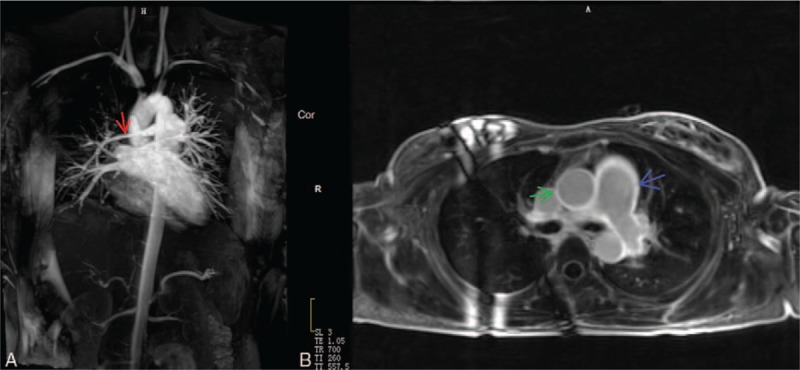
A 22-year-old woman with the active Takayasu arteritis. Magnetic resonance angiography (MRA) (A) showed the stenotic right pulmonary artery (red arrow). Delayed contrast-enhanced magnetic resonance imaging (DCE-MRI) with three-dimensional inversion recovery Turbo fast low-angle shot (3D IR Turbo FLASH) (B) showed delay enhancement in aorta (green arrow) and pulmonary arteries (blue arrow).
